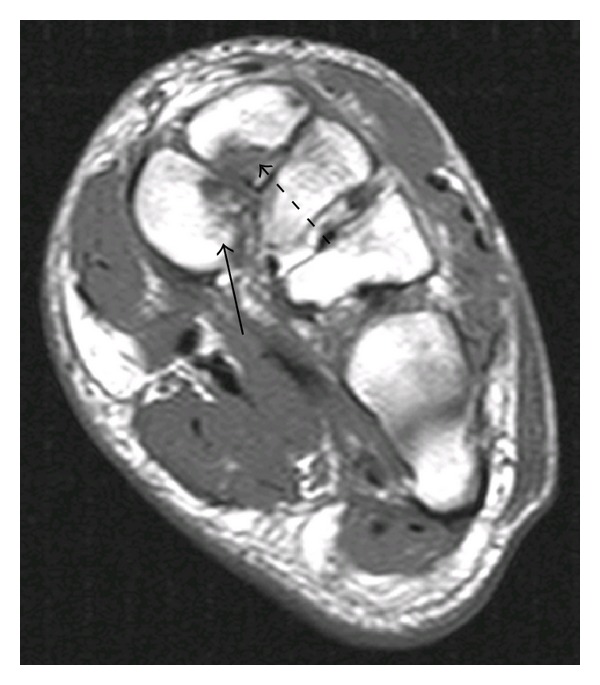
Coronal T1 MR image at the level of the midfoot shows a Lisfranc fracture of the medial cuneiform. The plantar Lisfranc ligament (solid arrow) and the Lisfranc ligament proper (dashed arrow) are demonstrated.
